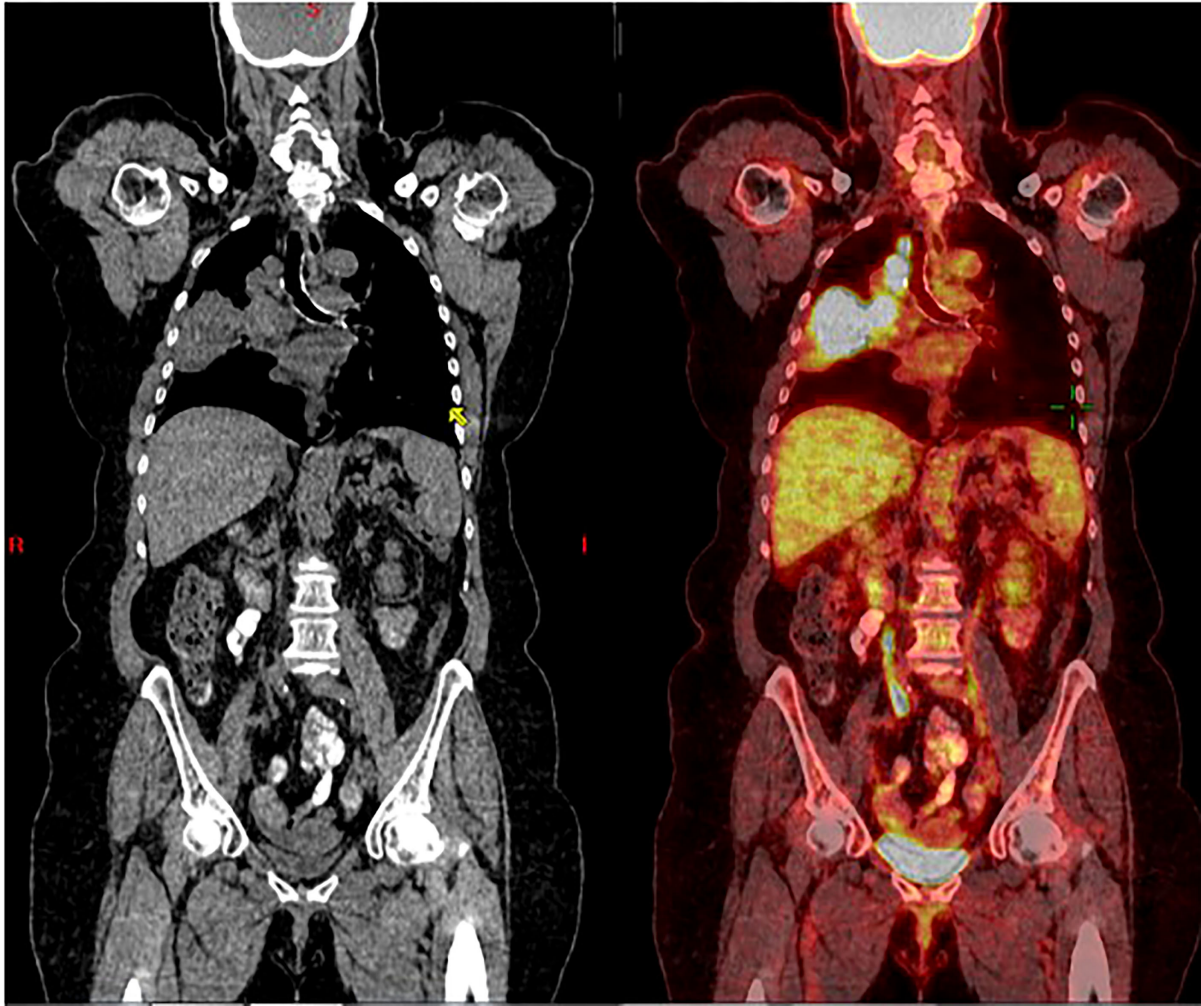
Coronal CT scan (left), and superimposed PET scan (right), from March 2016, show a large right lung mass (>5 cm) with increased uptake of FDG-18. There is also ipsilateral hilar and paratracheal lymphadenopathy with positive PET uptake, contributing to classification as unresectable, stage 3B NSCLC.
